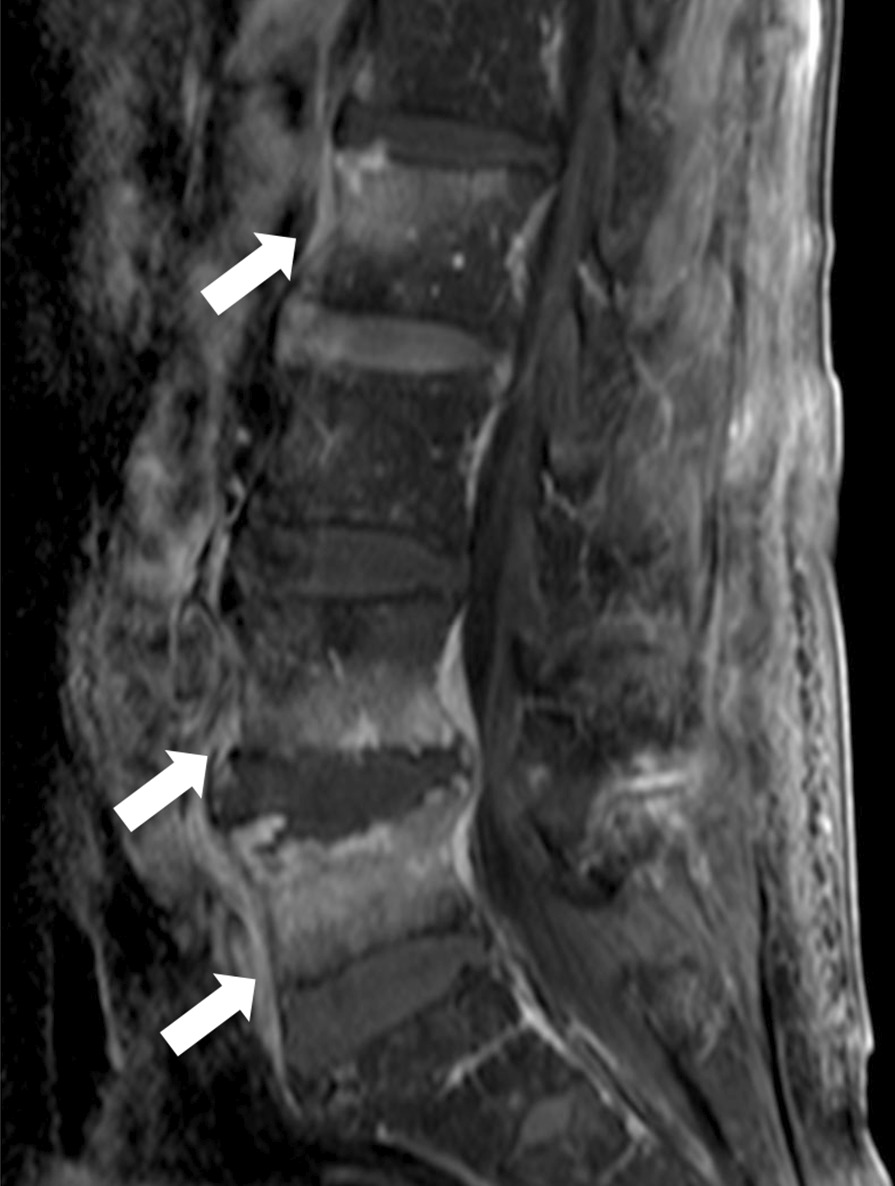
Lumbar images obtained 40 days after prostate biopsy. Contrast-enhanced T1-weighted magnetic resonance image of the lumbar spine shows contrast-enhancement of the L2, L4, and L5 vertebral bodies
